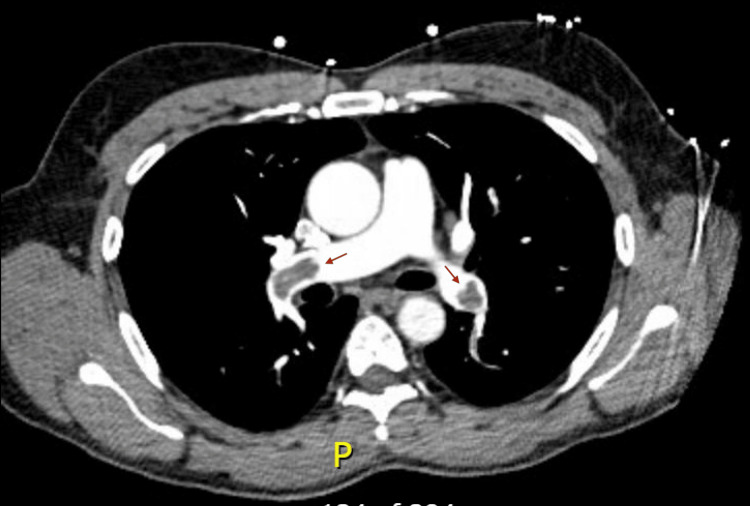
Representative image of the patient’s pulmonary emboli (marked with arrows) as seen on CT angiogram. The full study demonstrated extensive PE in the bilateral distal main, lobar, segmental, and subsegmental arteries
